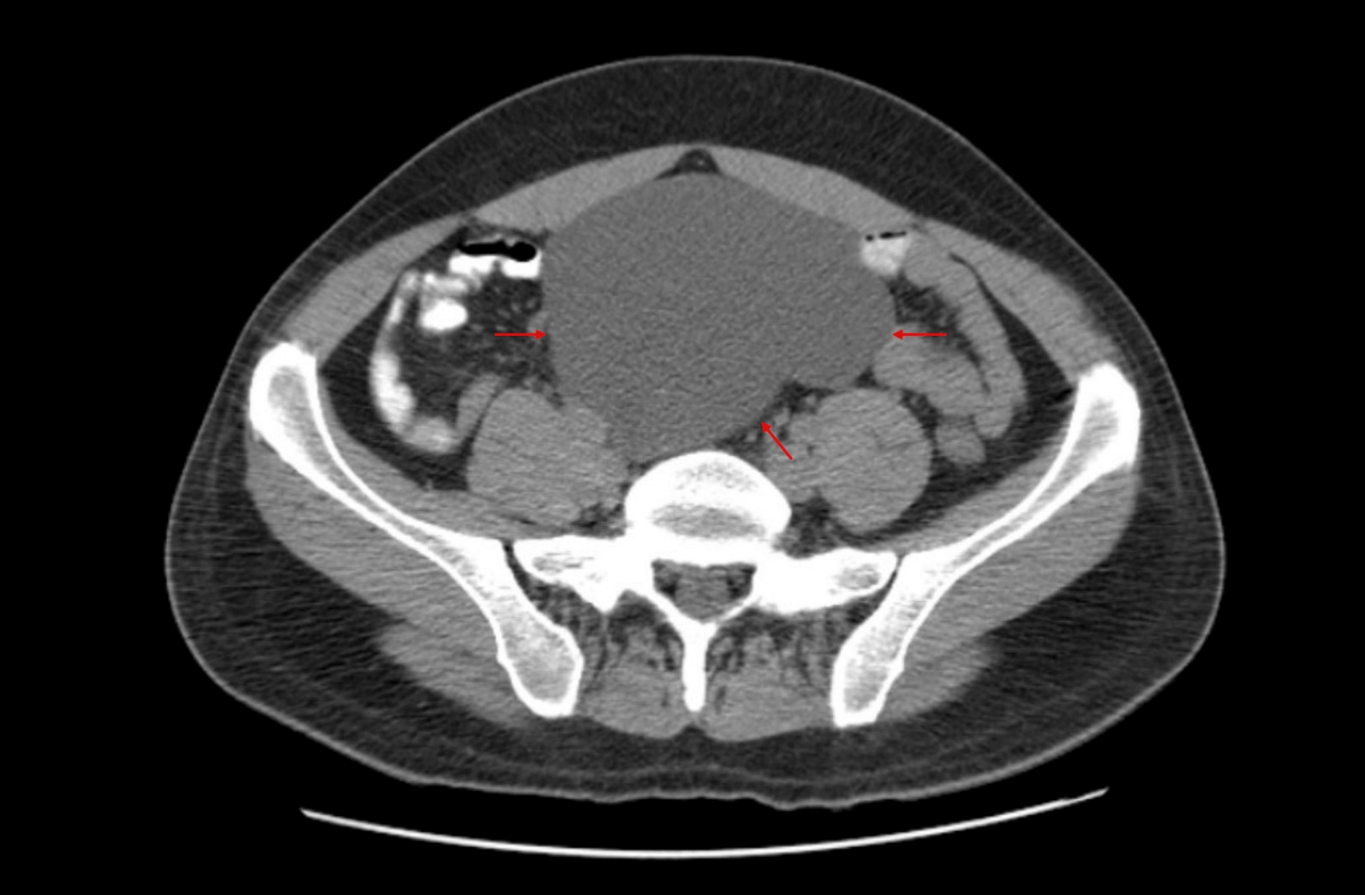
Contrast-enhanced CT scan, axial view, showing a mesenteric cystic mass with homogeneous liquid isodensity and wall enhancement after contrast administration; measures 11.9 cm × 9 cm × 20 cm CT: computed tomography
